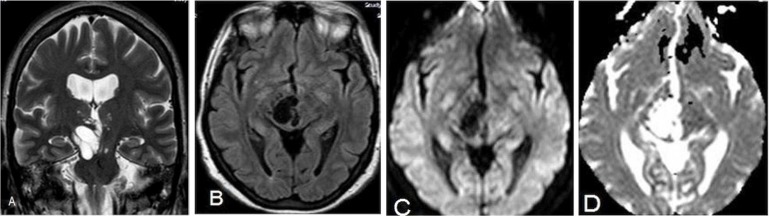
Coronal T2-weighted (W) (A) and axial fluid-attenuated inversion recovery (B) sequences magnetic resonance (MR) images demonstrate multilobular cystic mass isointense to cerebrospinal fluid in the mesencephalothalamic region. Coronal T2-W (A) MR image reveals the mild focal mass effect upon the third ventricle. Diffusion-weighted image (C) and apparent diffusion coefficient maps (D) show no restricted diffusion
